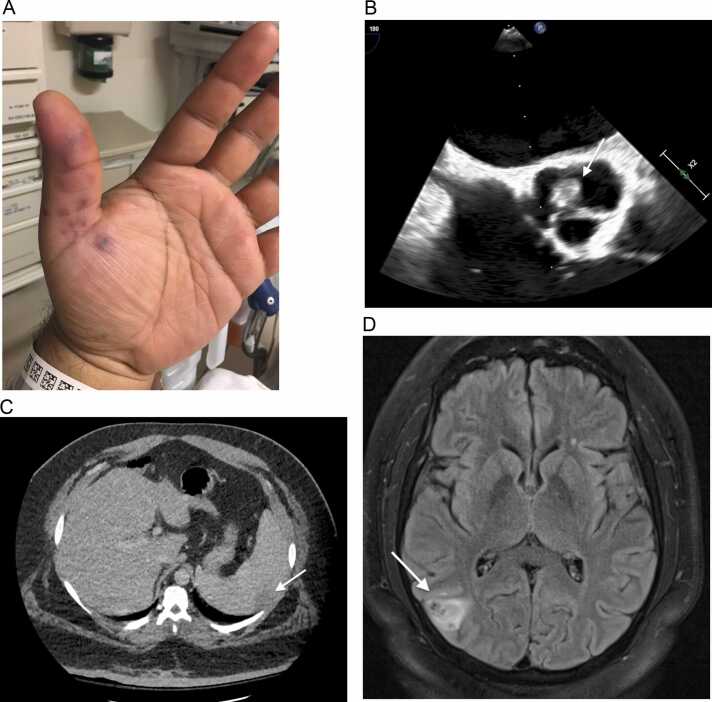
A. Osler’s Nodes in left thumb extending into palm. B. Transesophageal echocardiogram showing aortic valve vegetations. C. Wedge shaped area of decreased attenuation concerning for splenic infarction. D. Small acute to subacute infarction in the right occipital lobe, likely related to embolic focus. Small associated focal hemorrhage at this site is also seen.
